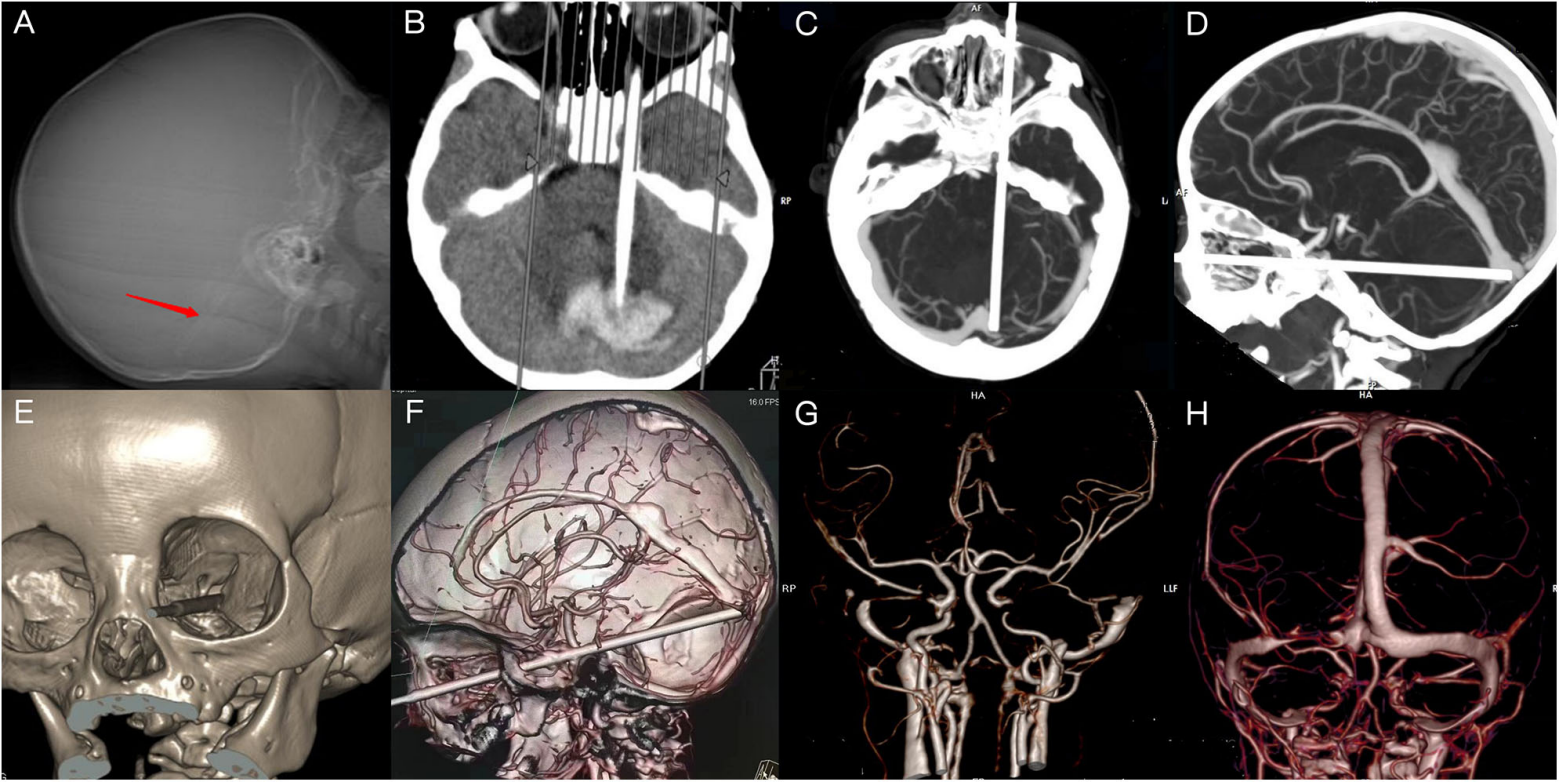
Imaging of X-ray and computed tomography (CT). (A) X-ray indicating the presence and position of a linear foreign body (FB). (B) CT revealing a strip-shaped focus and high-density areas in the adjacent areas. (C,D) The FB had pierced the left orbital apex and compressed the left transverse sinus. (E,F) The FB had penetrated the superior orbital fissure and cavernous sinus, in close proximity to the internal carotid artery (ICA). (G) Discontinuity of the cavernous segment of ICA. (H) Discontinuity in a portion of the left transverse sinus.
